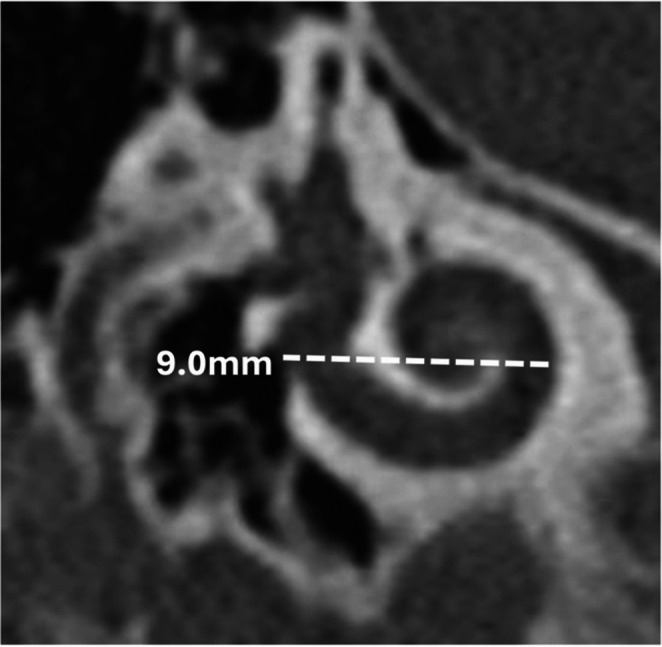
Cochlear diameter. Oblique sagittal CT demonstrating measurement of the cochlear diameter (dashed line), defined as the distance from the centre of the round window to the opposite outer wall of the basal turn. Cochlear duct length can be estimated based on cochlear diameter, which is important for guiding electrode selection and insertion length
